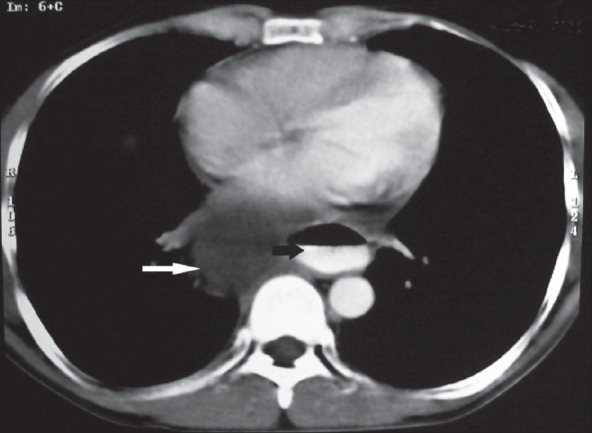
CT scan of lower chest after oral and IV contrast showing a cystic lesion in posterior mediastinum compressing the esophagus. Air contrast level is seen in lower esophagus suggestive of stasis
